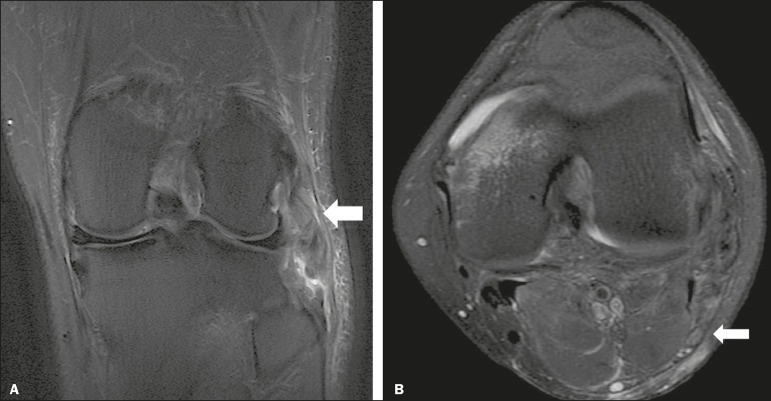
A 21-year-old male patient who was in a motorcycle accident. A: Coronal T2-weighted MRI sequence with fat saturation, showing injuries to PLC structures (arrow). Note the complete tears in the LCL and biceps femoris tendon, as well as the partial tears in the popliteal tendon and arcuate ligament. B: Axial T2-weighted sequence with fat saturation, showing the CPN with signal alteration but without discontinuities (arrow), which was interpreted as axonotmesis.
